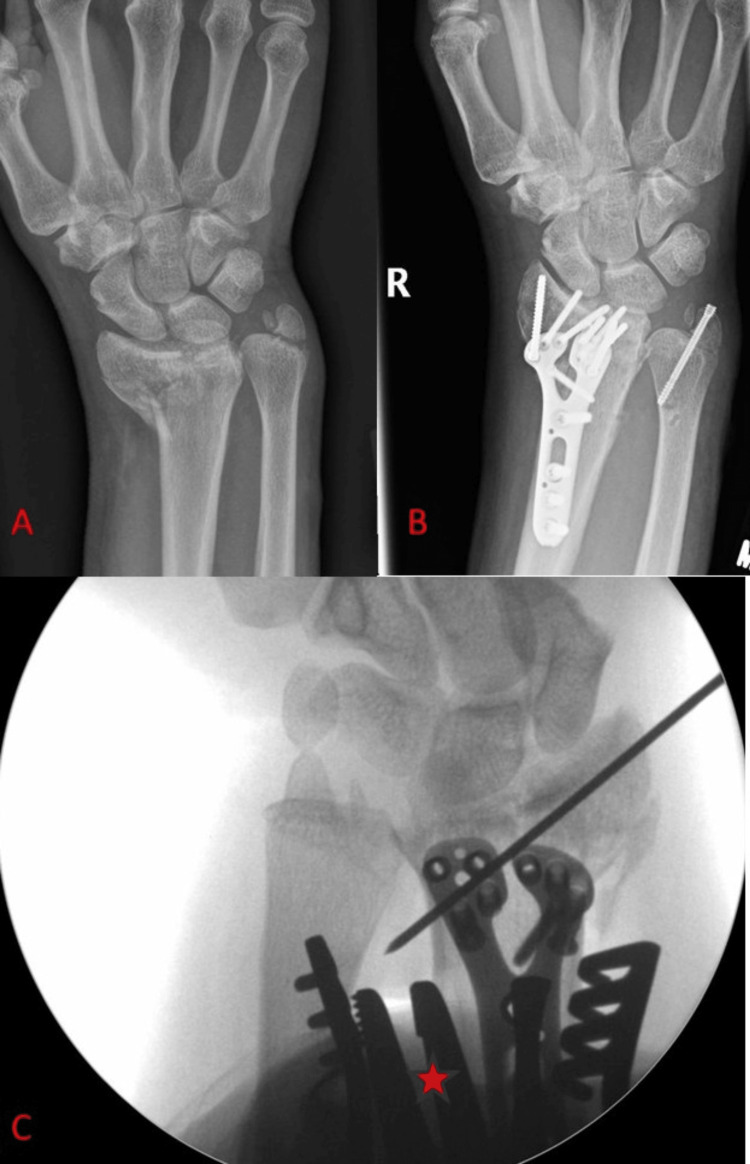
Case example where a laminar spreader is placed between the radial and ulnar shafts. (A) Anteroposterior radiograph showing significant displacement and comminution of the distal radius fracture. Note the collapse of the lateral column, resulting in radial tilt of the distal fragment. (B) Anteroposterior radiograph showing the near-complete correction of the previous deformity after the intraoperative use of the laminar spreader. (C) Intraoperative radiograph of a different patient, showing appropriate placement of the blades of the laminar spreader between the distal radial and ulnar shafts, noted with a red asterisk.
